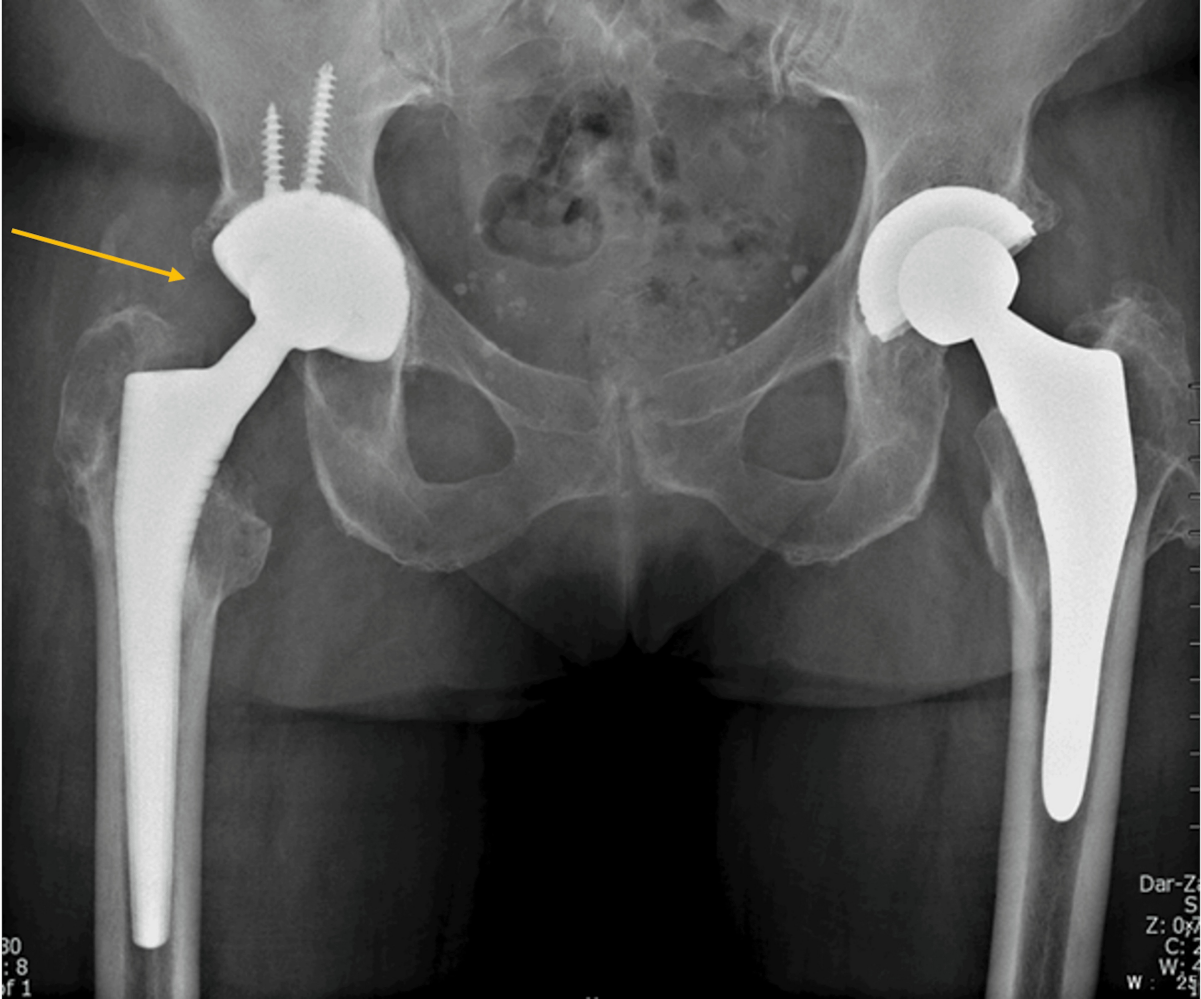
Anteroposterior pelvic X-ray six weeks after right revision total hip arthroplasty using a dual-mobility construct and a cementless stem.
